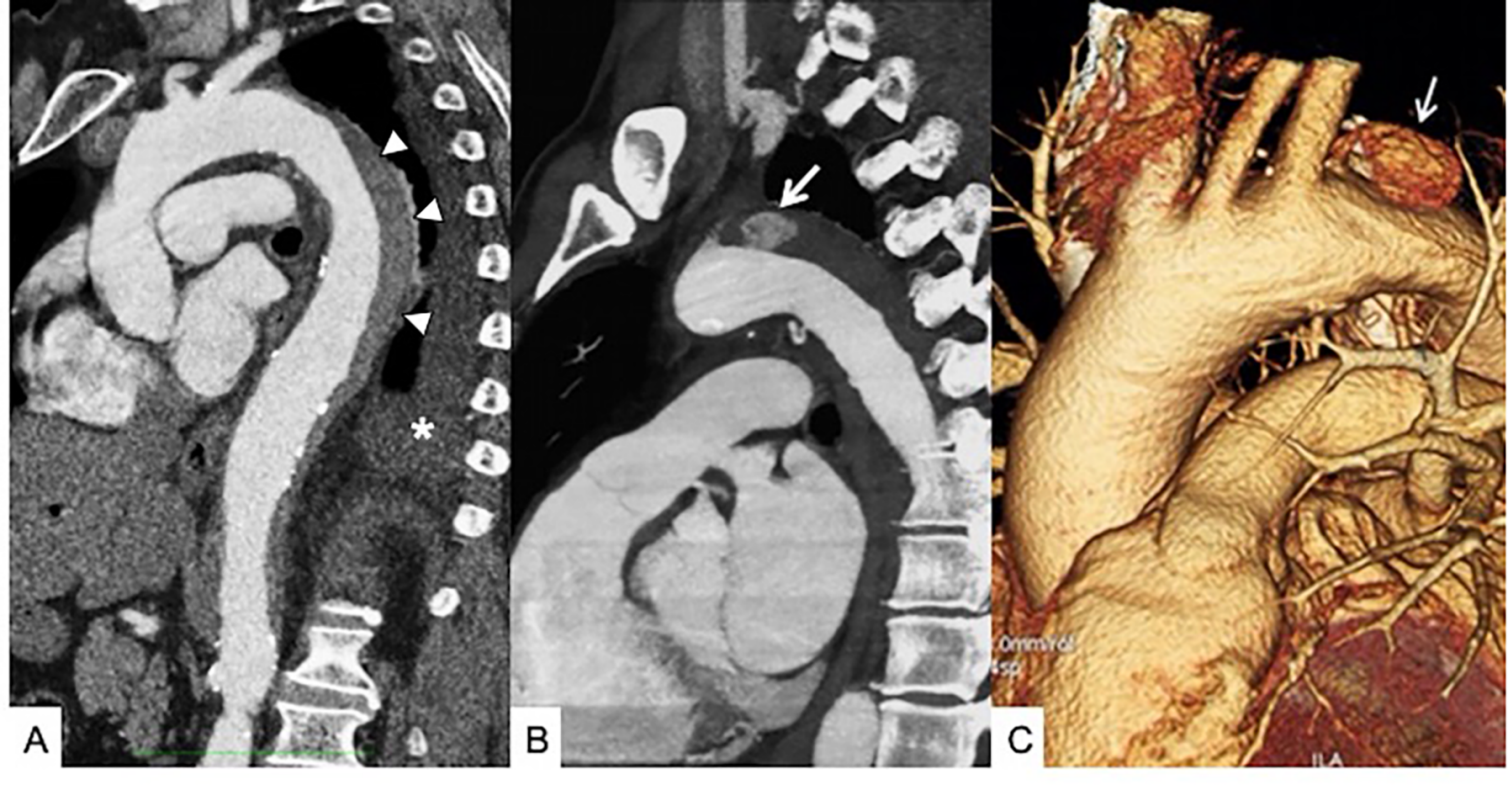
Unstable Type B IMH. (A) Sagittal contrast-enhanced baseline CT shows Type B IMH (arrowheads) in a 68-year-old male patient with hypertension peak and persistent thoracoabdominal pain; pleural effusion is present (asterisk). (B) Six-day follow-up contrast-enhanced CT sagittal multiplanar reformation shows the appearance of an ULP close to the aortic isthmus (arrow). (C) Sagittal oblique 3D volume rendering (VR) reconstruction confirms ULP (arrow). The patient undergoes TEVAR.
